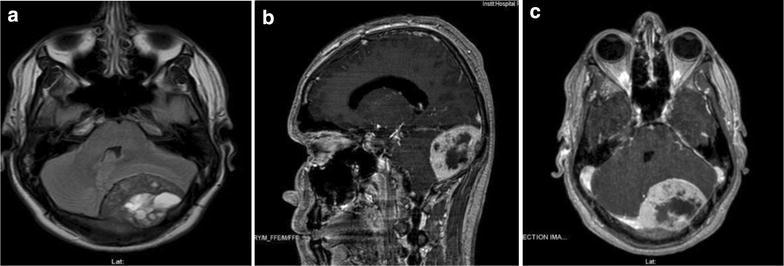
Preoperative MRI images. a FLAIR axial, shows an infratentorial tumor that deforms the fourth ventricle, with hiperintense areas that suggest hemorrhage. b, c T1 with gadolinium enhanced axial and sagital, showing irregular enchantment of the tumor
